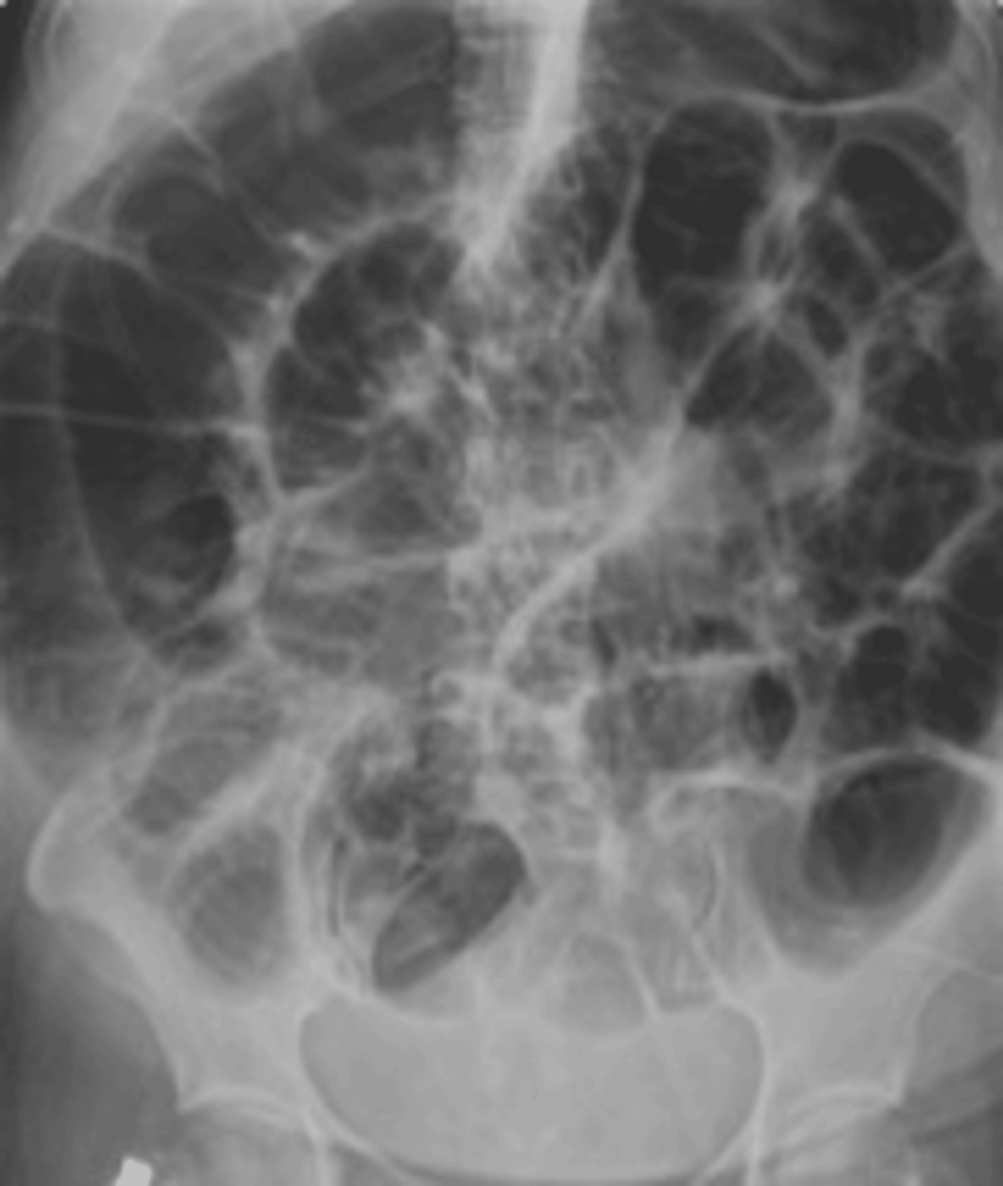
Abdominal radiograph showing diffuse colonic dilatation, most prominent in the cecum, consistent with acute colonic pseudo-obstruction (Ogilvie's syndrome).
